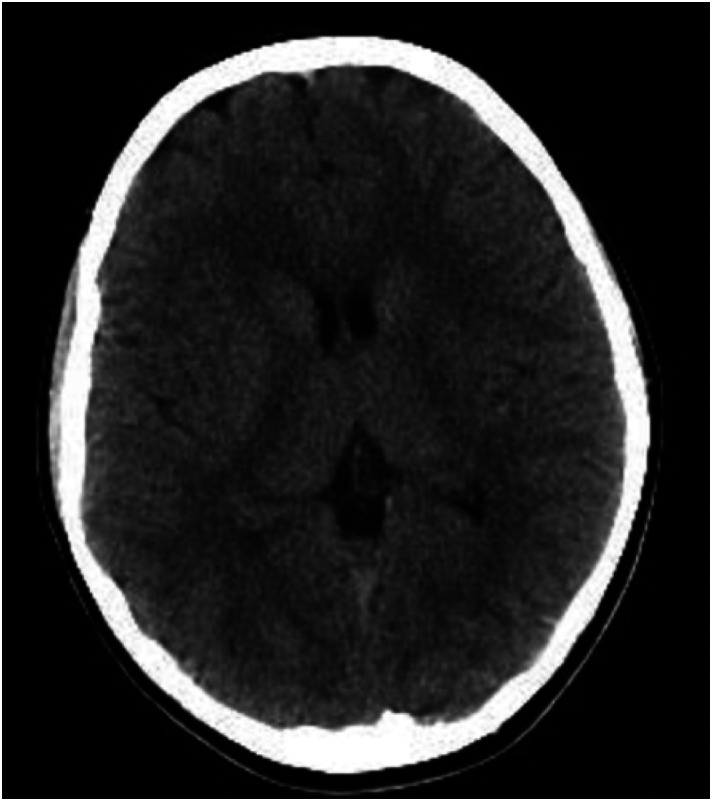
Normal non-contrast computed tomography of the brain.
